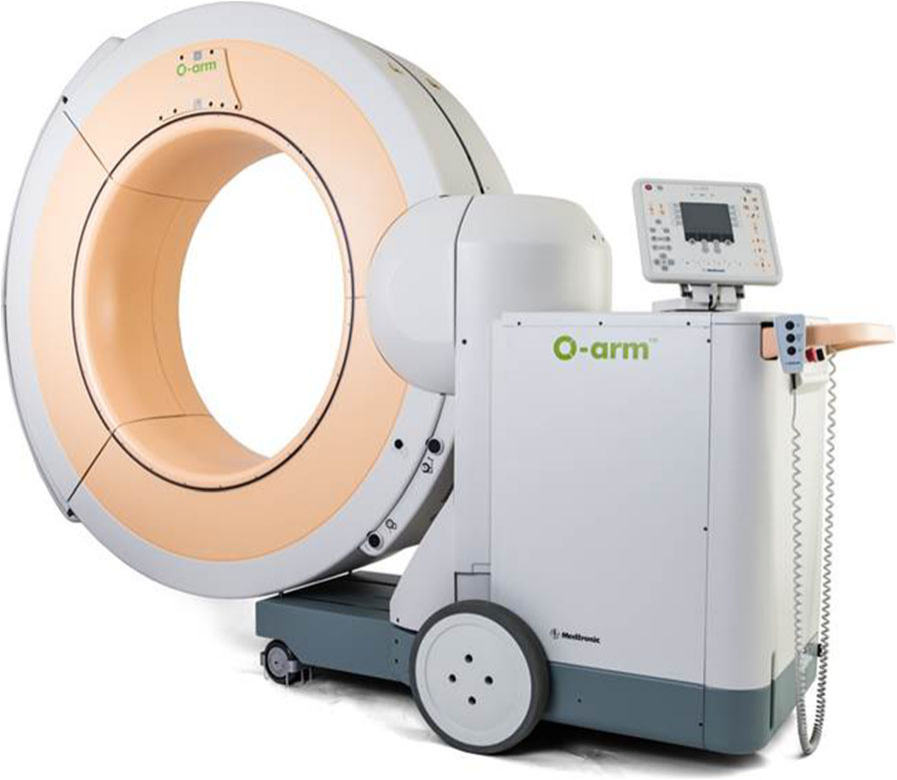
The O-arm mobile surgical cone beam CT imaging device (Medtronic GmbH, Earl-Bakken-Platz 1,40670 Meerbusch, Germany)
